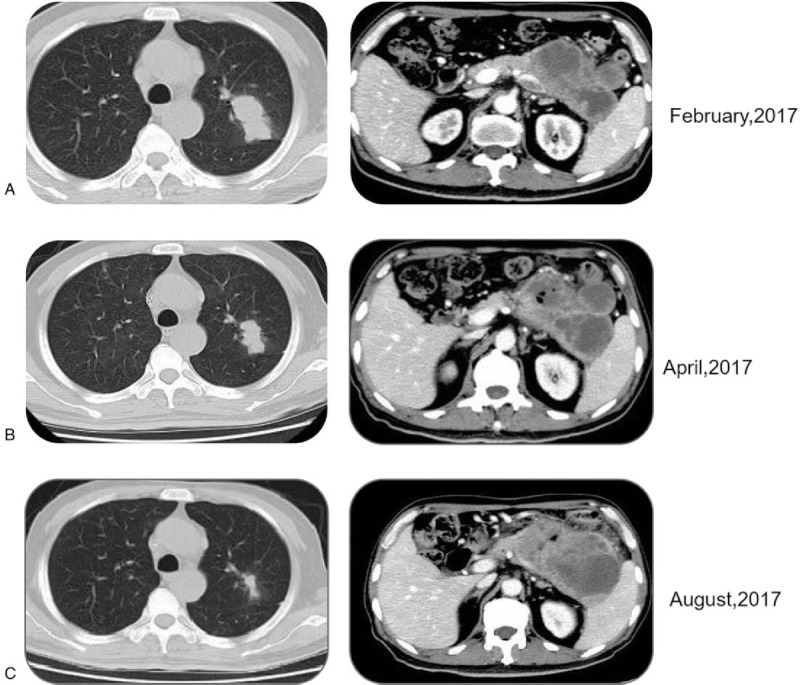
CT scans at baseline of AG treatment (A), after 2 cycles of AG (B), and after 6 cycles of AG (C).
